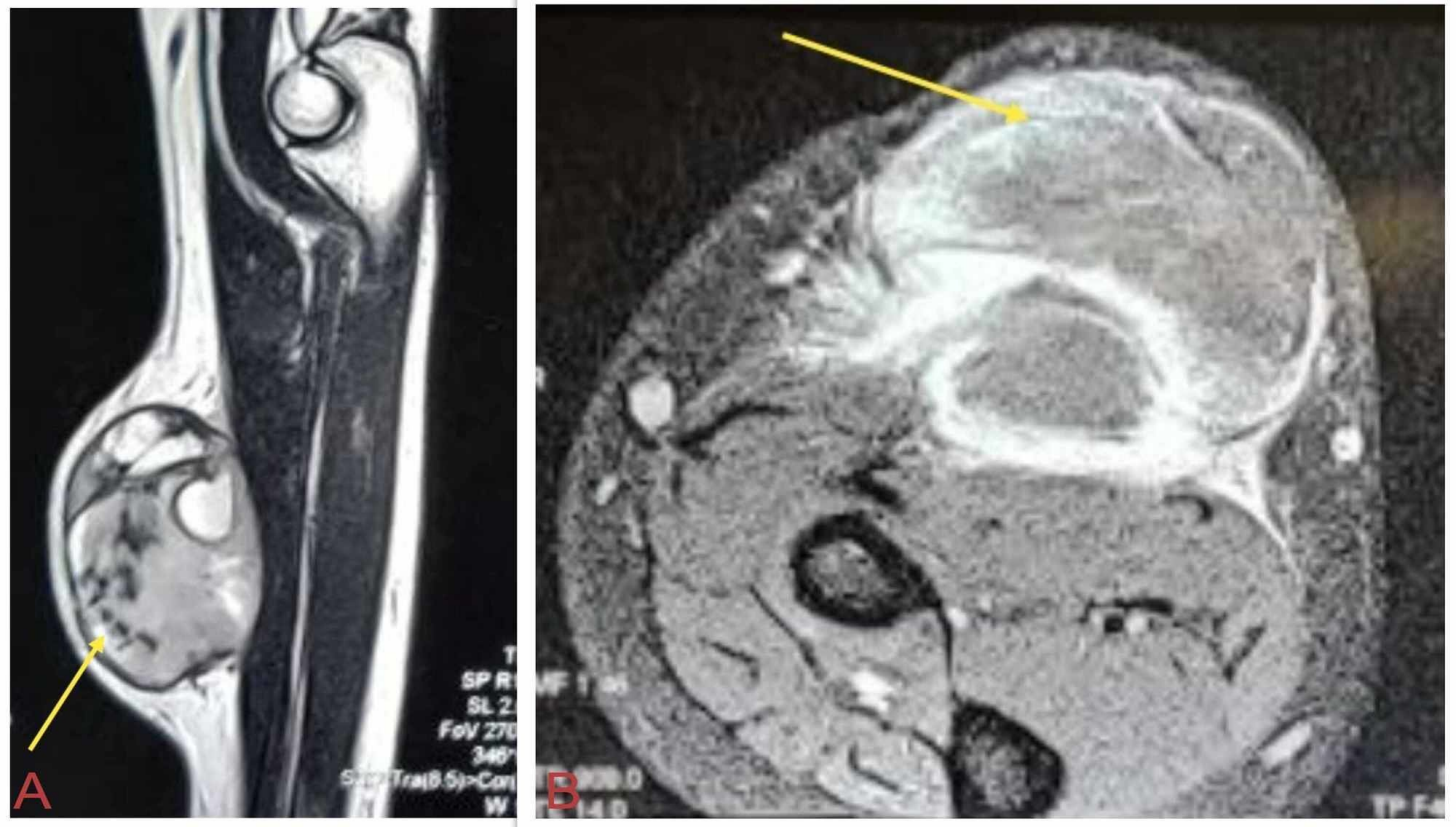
MRI shows subcutaneous enhancing soft tissue lesion with internal hemorrhagic and necrotic components involving the flexor carpi radialis muscle. (A) Sagittal section. (B) Transverse section.
